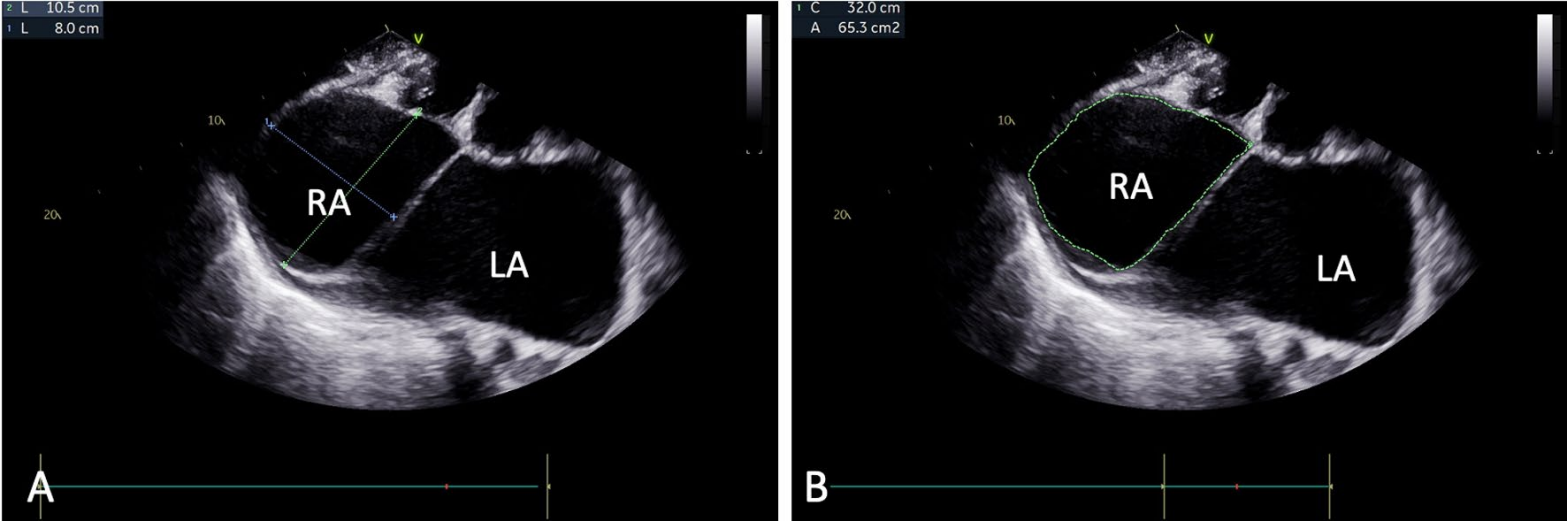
Two-dimensional echocardiography shows giant right atrium with dimensions of 10.5 × 8.0 cms (A) and area of 65.3 cm^2^ (B)
